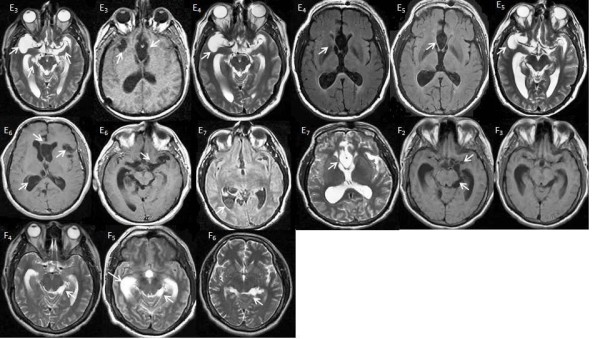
MRI of patients. E3 (MRI T2, T1): Parasites in Sylvian, basal and lamina terminalis cisterns. E4 and E5 (MRI T2, FLAIR): Persistence of some of the parasites. E6 (MRI simple, CE T1): Persistence of lamina terminalis parasites and appearance of new ones. E7 (MRI FLAIR, T2): Persistence of parasites. F2 (MRI T1): Parasites in the crural and ambient cisterns. F3 (MRI T1), F4, F5 and F6 (MRI T2): Persistence of ambient cistern parasites.
